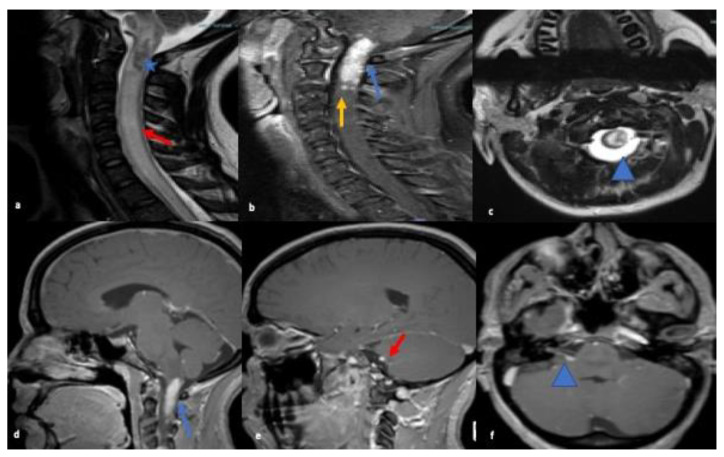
MRI sagittal T2 images (a) of the cervical spine reveal ill-defined long segment signal alteration with prominent cord expansion (red arrow) and ill-defined heterogenous lesion in cervicomedullary junction of the cord (blue asterisk); and (b) T1 post contrast image shows cord enhancement (blue arrow) and multiple separated subtle foci of enhancement were noted at C3 (yellow arrow). Axial T2-weighted (c) reveals corresponding hyperintense cord signal (blue arrowhead). MRI Brain sagittal and axial T1 post contrast images highlights homogenous enhancement at cervicomedullary junction (blue arrow; (d)), and enhancement along CN VII and VIII nerve complex (red arrow; (e)) & (blue arrowhead; (f)).
